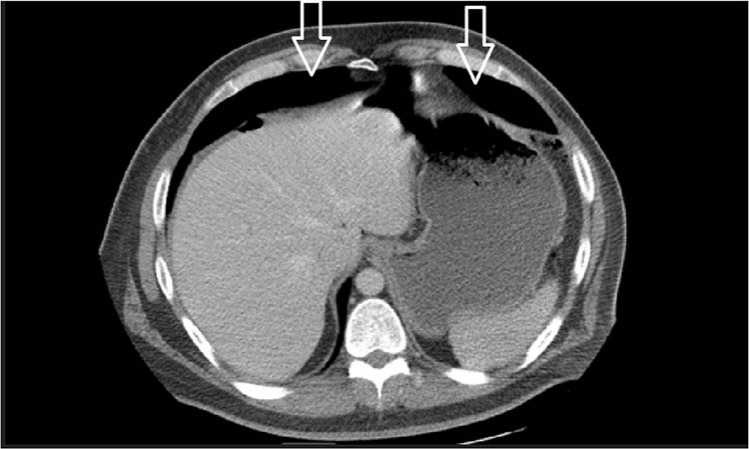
Similar appearance of moderate volume pneumoperitoneum compared with last CT.
